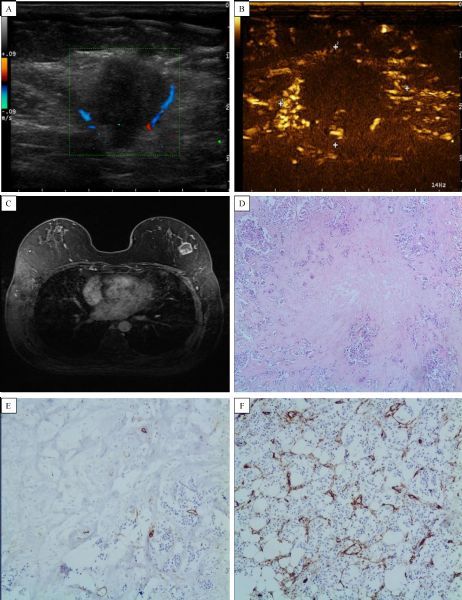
Infiltrating ductal carcinoma examination by contrast-enhanced ultrasound. A: Peripheral blood flow could be seen, and internal blood flow was not obvious, with a lesion size of about 20 mm × 16 mm. B: Peripheral radiate enhancement could be seen, with internal enhancement slightly low and uneven. C: Enhanced MRI data for the same patient showing a peripheral ring and slightly low internal enhancement. D-F: Pathology data showing internal necrosis and collagenization (CD34 indicated a small collagenization region in the tumor center); small vessels in the tumor periphery were more dense than in the tumor center region (upper figure). Sizes were consistent in contrastenhanced ultrasound, enhanced MRI, and postoperative pathology. Pathological data showed infiltrating ductal carcinoma, grade Ⅱ-Ⅲ.
